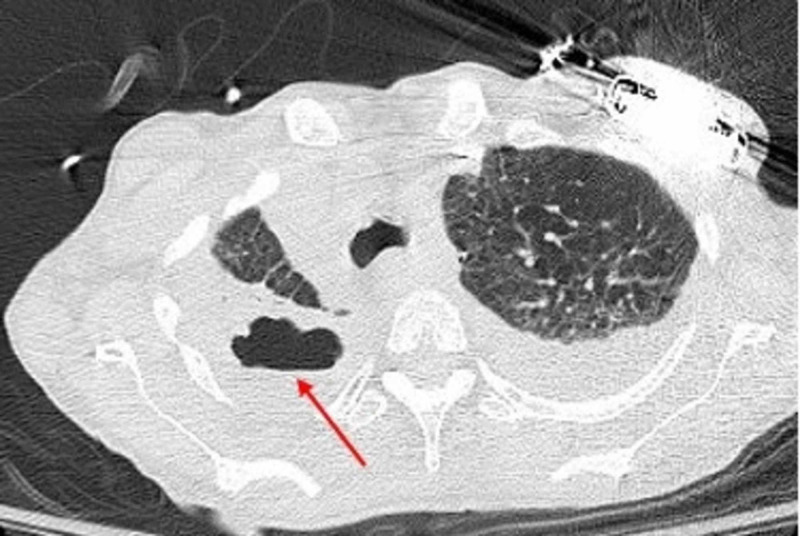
CT-thorax obtained one week after treatment initiation showing stable right upper lobe cavitary lesion (red arrow) with surrounding consolidation
